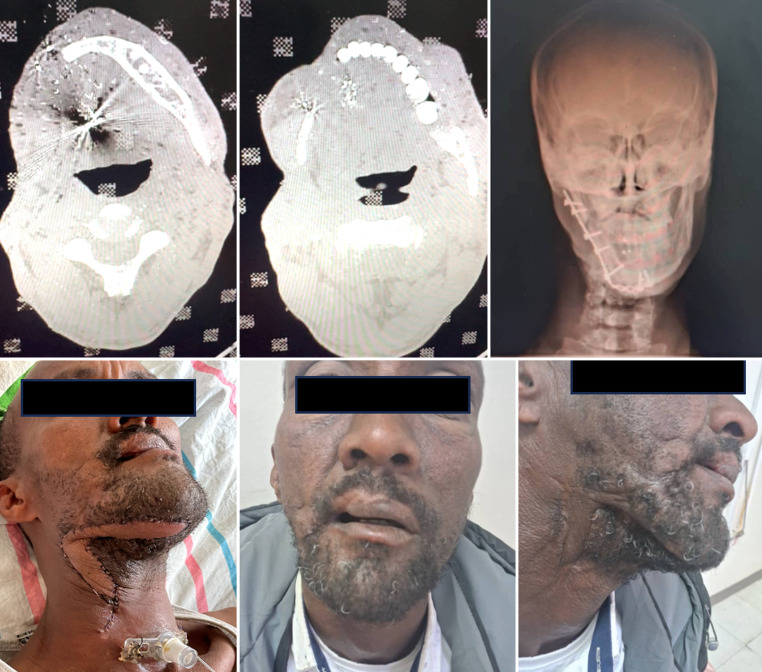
Case 1: secondary mandibula reconstruction after gunshot injury. a, b Preoperative CT scan of the mandible, showing the segmental defect after shotgun injury. c Postoperative X-ray confirming fixation of the free fibula flap with reconstruction plate (a postoperative CT was not performed due to limited availability and high costs for patients in Africa). d-f Postoperative clinical photograph, showing restored mandibular contour and demonstrating soft-tissue healing
